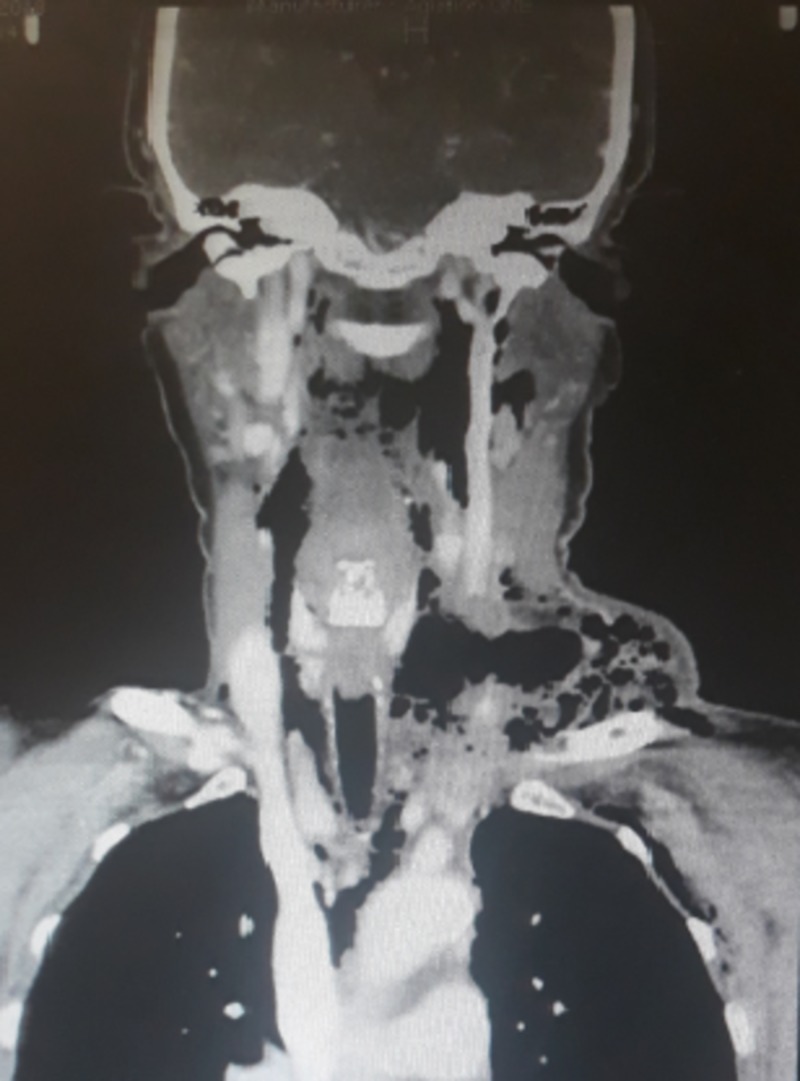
Coronal view of contrasted computed tomography scan of the neck and chest showing diffuse gas collection in the neck and left supraclavicular region extending into the mediastinum
